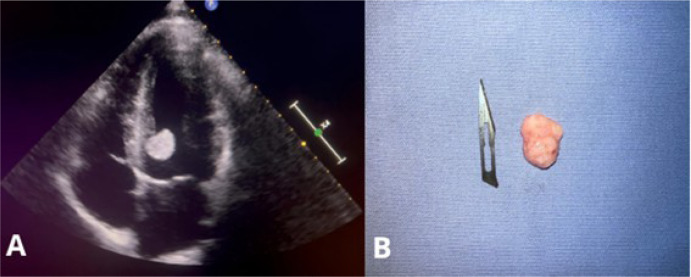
(A) Transthoracic echocardiogram revealing a mass in the left
